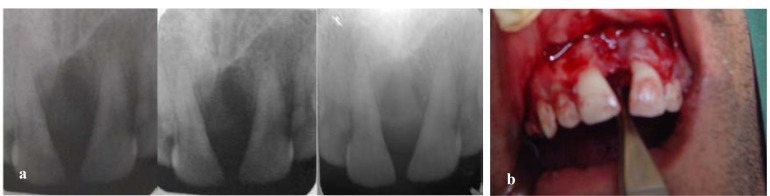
Periapical radiograph after six month follow- up. There were no findings of recurrence b There was no sign of recurrent after six months follow-up
